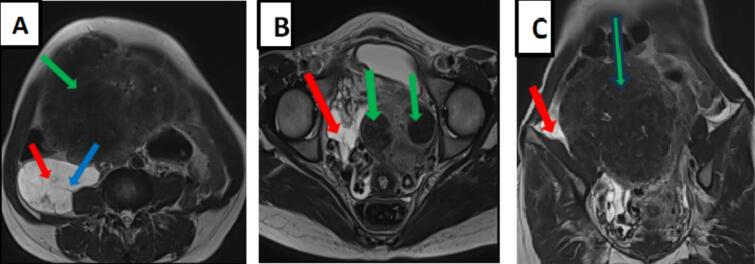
Axial sections (A,B) and a coronal section (C) on T2-weighted pelvic MRI showing an abdominopelvic cystic (red arrow) molding adjacent structures containing thin septa (blue arrows) associated with a polymyomatous uterus in T2 hyposignal (green arrow). (For interpretation of the references to colour in this figure legend, the reader is referred to the web version of this article.)
